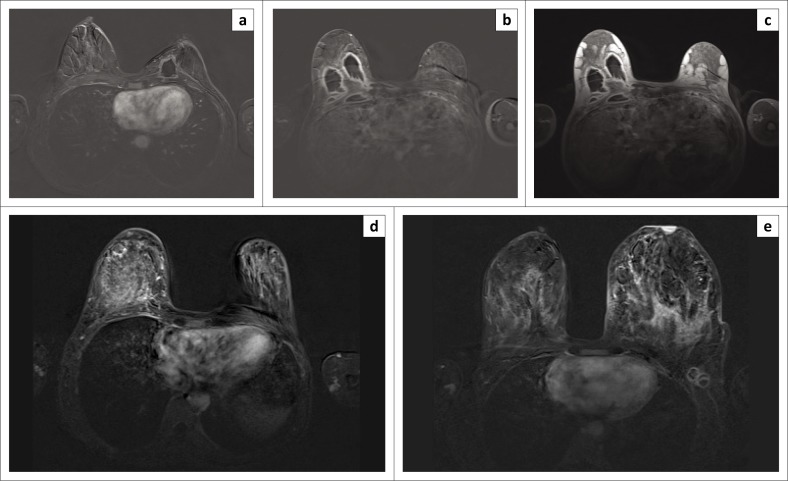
Dynamic contrast-enhanced magnetic resonance imaging enhancement patterns of various patients with breast tuberculosis: (a) focal rim, (b) multiple regions rim, (c) same patient as (b) to better demonstrate multiple rim enhancement on the non-subtracted T1-weighted image, (d) clumped diffuse and (e) clumped diffuse associated with rim-enhancing left axillary nodes.
